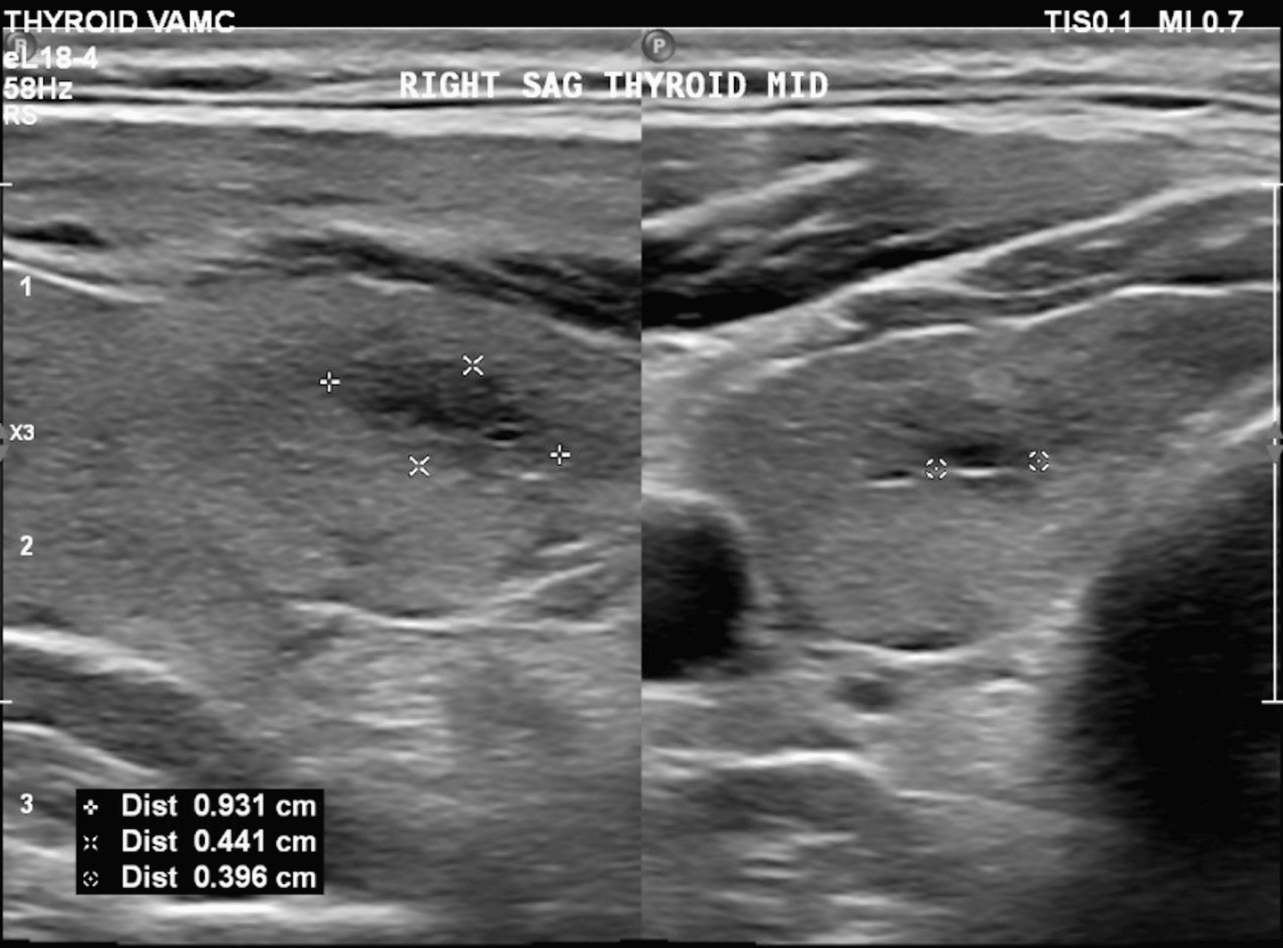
Sagittal mid-right thyroid ultrasound image demonstrating a subcentimeter, ill-defined, mildly hypoechoic area measuring 0.3 × 0.4 × 0.9 cm, without calcifications or taller-than-wide morphology, favored to represent a pseudonodule in the setting of heterogeneous thyroid parenchyma.
